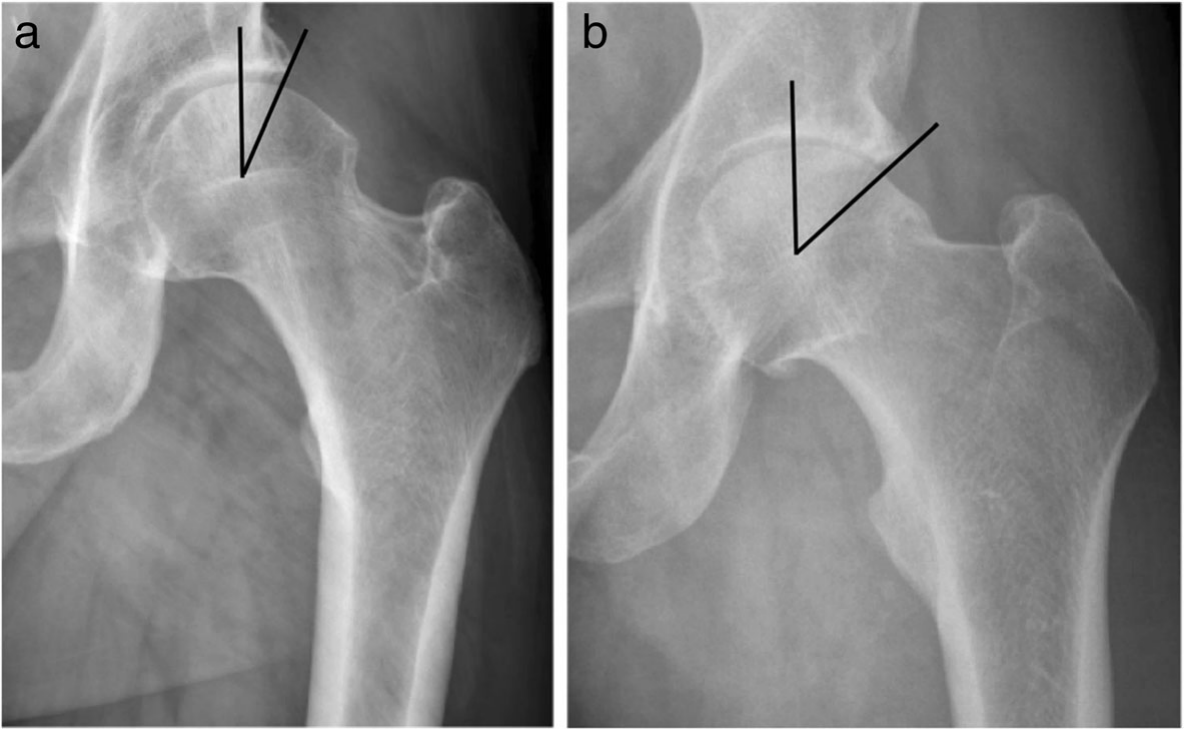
The figure below contains a side-by-side comparison of preoperative anteroposterior pelvis radiographs used in this analysis. The radiograph on the left a demonstrates a normal lateral center-edge angle (LCEA) of Wiberg (< 40°), and the radiograph on the right b demonstrates a pincer deformity (LCEA ≥40°). Military veterans had significantly greater LCEAs compared with those of civilians using an independent t-test (*P* = 0.04). Military veterans also demonstrated a significantly greater prevalence of pincer deformities when compared with civilians using Fisher’s exact tests (*P* = 0.025)
